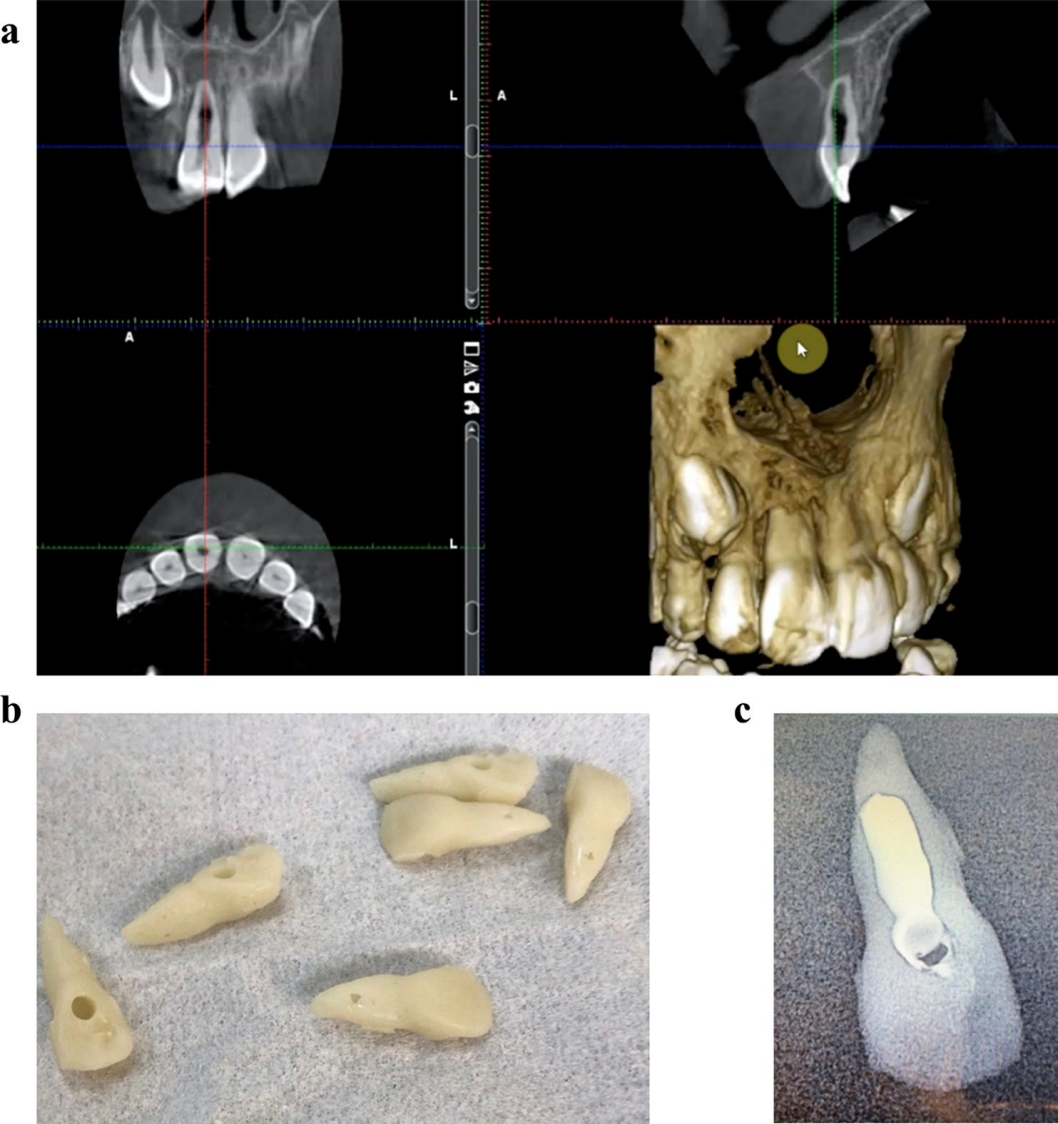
(a). High-resolution CBCT scan showing the internal resorption defect. (b). 3D printed teeth with virtual access cavity. (c). Calcium hydroxide paste injected and radiographed to check the radiographic appearance of the resin material
